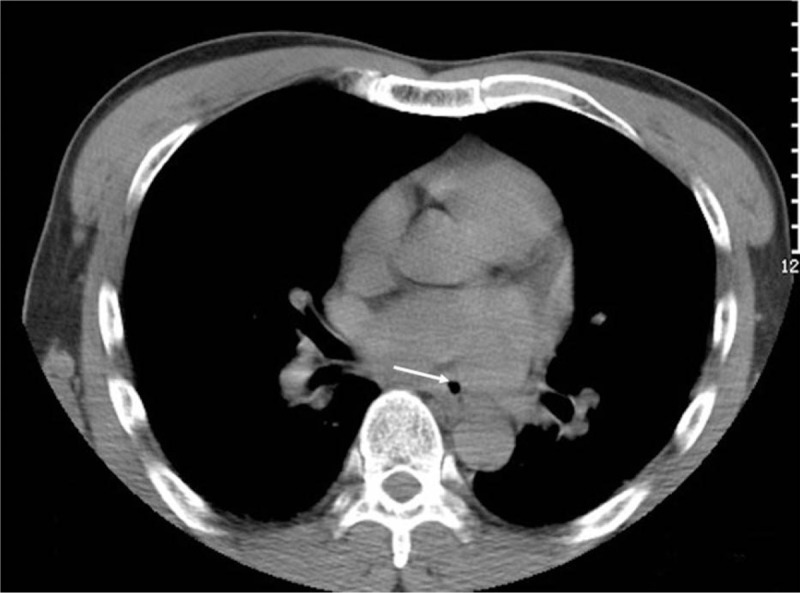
Chest CT suggested gas shadow in the middle segment of the esophagus near the left atrium, suggesting atrial esophageal fistula (as shown by the arrow).
